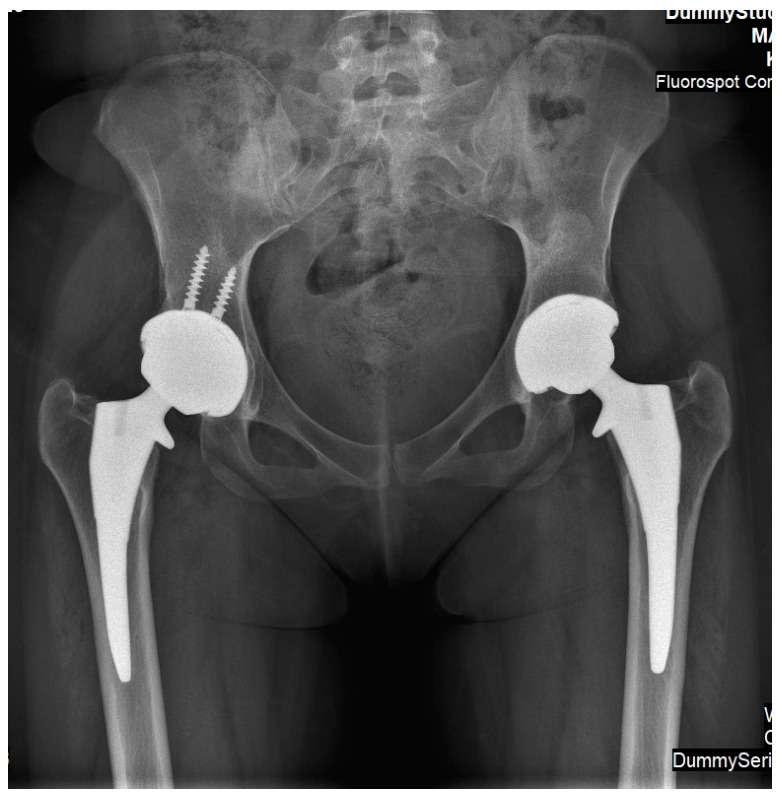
An antero-posterior X-ray of pelvis and hip joints of Case 2. It was performed after she underwent simultaneous bilateral hip arthroplasty. Implants are similar; however, acetabular cup on right side was augmented using two screws (based on surgeons’ preferences).
